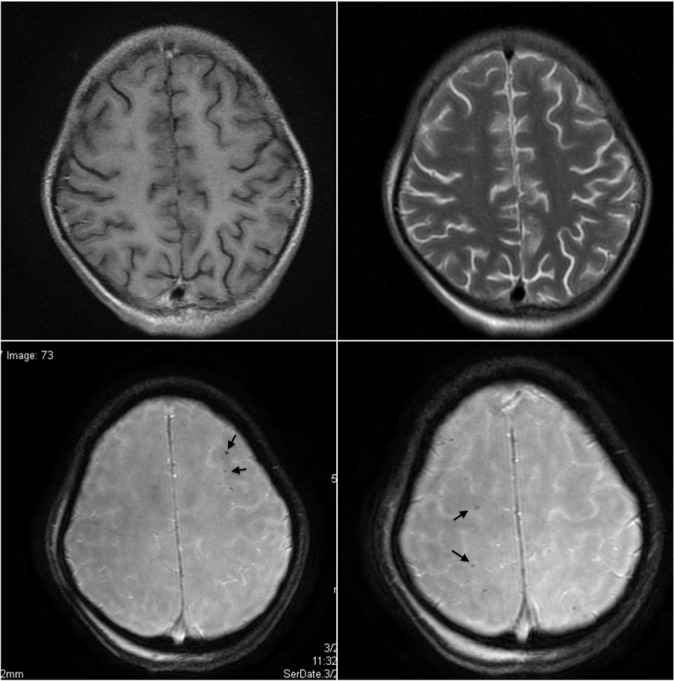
MRI BRAIN with T1 T2 (top left and right) and gradient echo (GRE, below) sequences. GRE sequences showing multiple foci of tiny punctate hemorrhage (Blooming on GRE; marked with arrow) in right fronto-parietal and left frontal regions.
